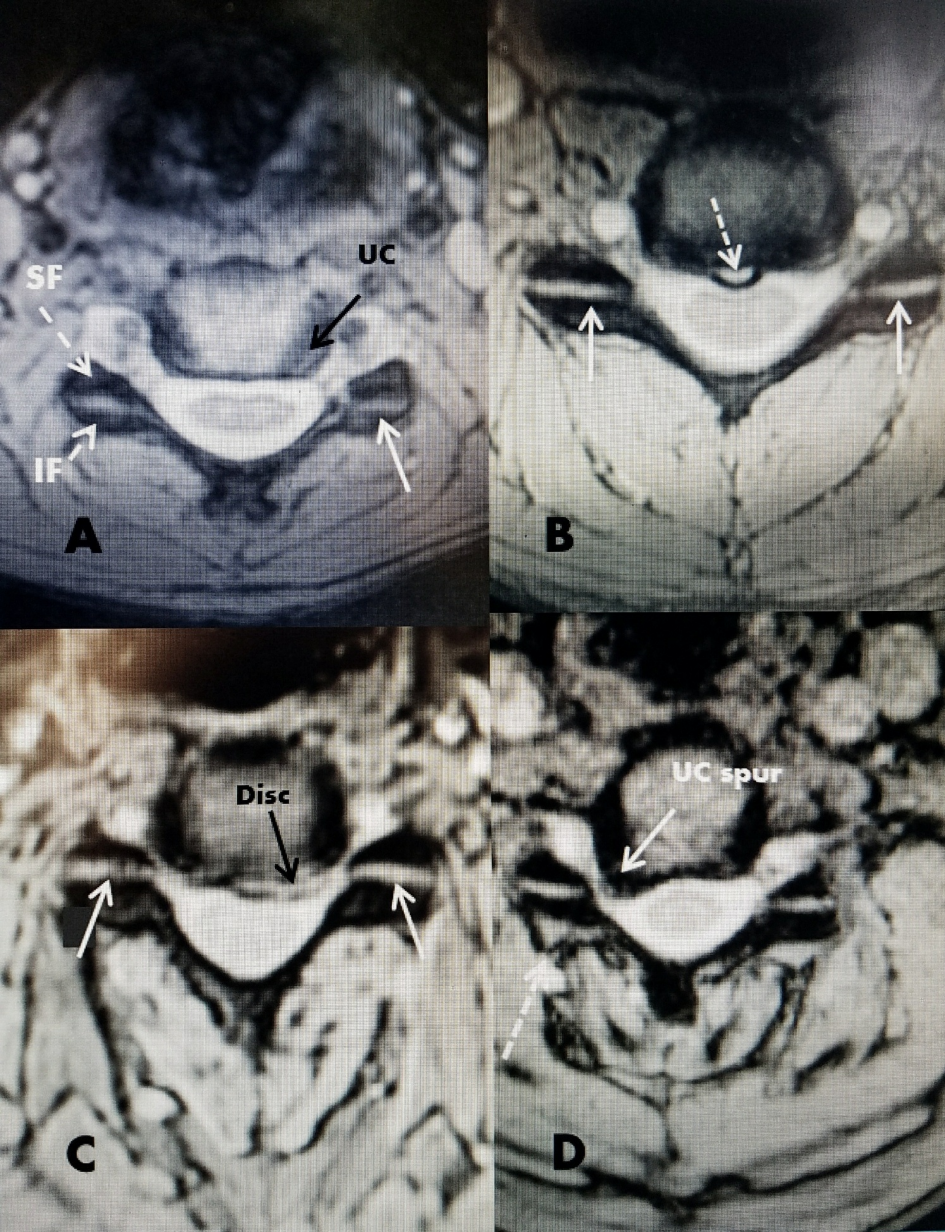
Axial magnetic resonance imaging (MRI) showing the relationship of the facet joint to other cervical structures A: Normal axial image of MRI cervical spine. The superior facet (SF) and inferior facet (IF) can be clearly seen. The uncovertebral joint (UC) can be seen lateral to the disc (solid black arrow). The uncovertebral joint emanates from the more lateral edge of the inferior vertebrae. The posteriorly located horizontal facet joint is seen (solid white arrow). B: Small midline annular tear (dotted white arrow). The facet joints are seen on both sides (solid white arrow). C: Herniated disc into the ventral floor of the cervical canal (solid black arrow). The facet joints are clearly seen (solid white arrow). D: Large posterior and lateral osteophyte from the back of the uncovertebral (UC) joint forming a spur into the intervertebral foramina (solid white arrow). The horizontal facet joint is clearly seen as in the other examples but there is minimal posterior enlargement of the facet capsule on the same side as the large osteophyte (dashed white arrow).
